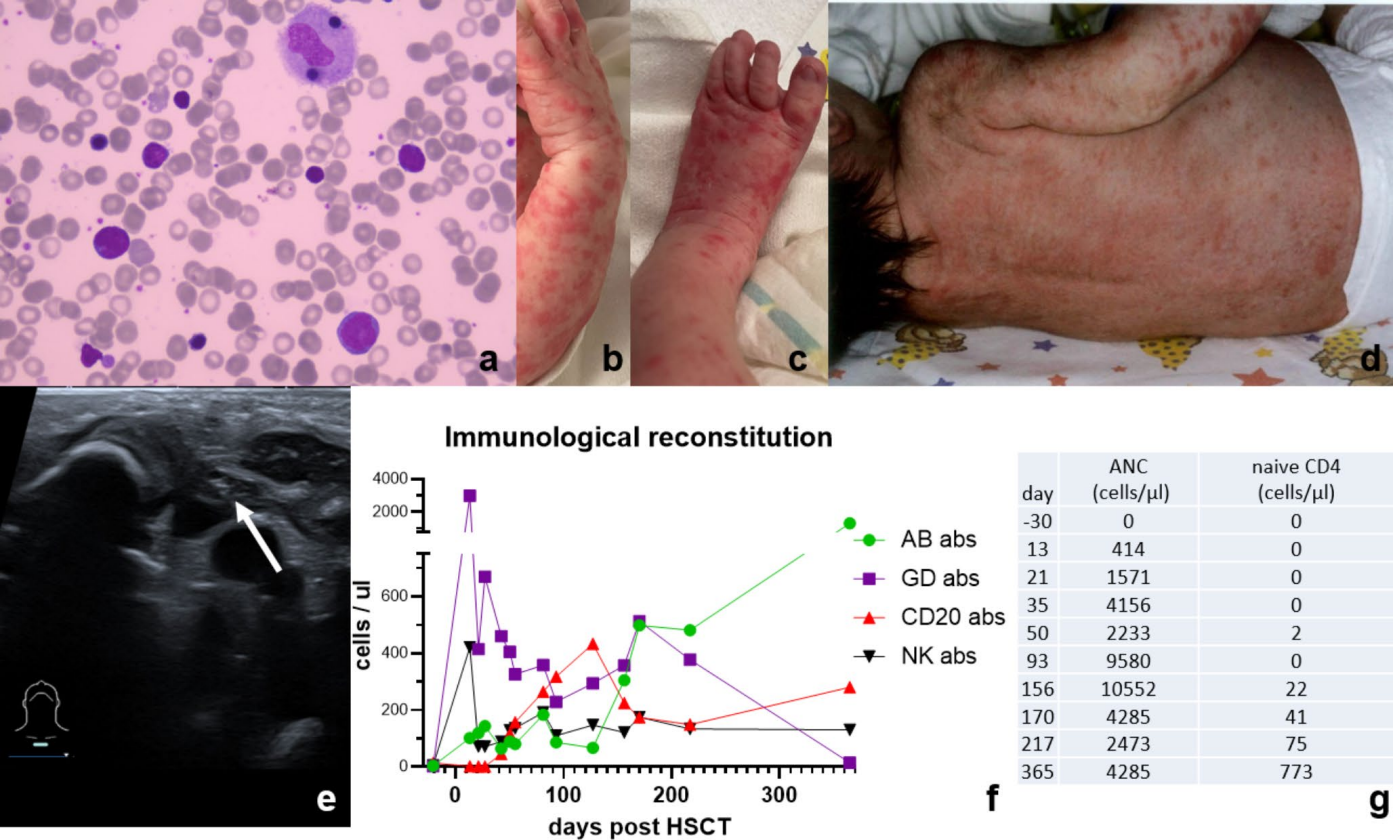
a. Bone marrow aspirate showing normal red blood cells and platelets. Few maturing lymphocytes are seen, however myeloid maturation is not beyond through the blast / promyelocyte stage, neutrophil granulocytes are absent. b-d. Skin manifestations after birth: maculopapular rash on trunk and distal upper and lower limbs as signs of maternal GvHD or bacterial superinfections e. Thymic ultrasound at admission indicating a small thymus (white arrow) remnant suggestive of severe combined immunodeficiency f. Immunological reconstitution after HSCT: The chart shows the course of the immunological reconstitution in our transplanted reticular dysgenesis patient. Left y-axis: CD3 gamma/delta (■), CD3 alpha/beta (●), CD20 (▲), and NK (▼) cell count. As known in TCRab/CD19 depleted haplo SCT the immediate CD3 increase reflects expansion of TCRgd T cells. The increase is notably higher than in other TCRab/CD19 haplo SCT performed in screened SCID patients. All cells are consistently of donor origin. g. ANC and naive CD4 cell count before and after transplantation
